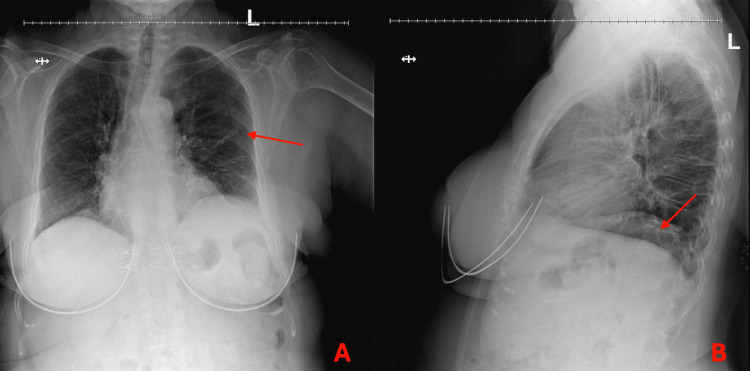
Chest X-ray A and B showing the anterior-posterior and lateral X-ray views of the chest, respectively. A shows left subsegmental atelectasis as indicated by the arrow. B shows a left lower lobe consolidation as indicated by the arrow
